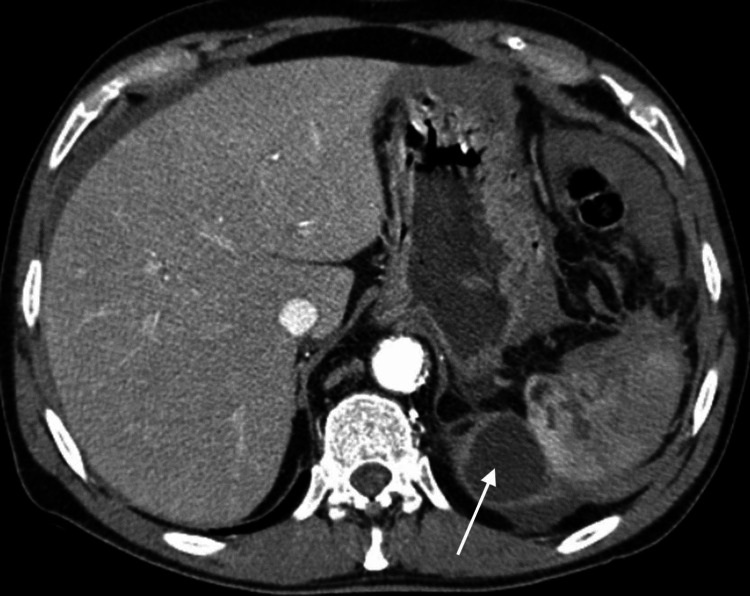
CT scan demonstrating an abdominal pancreatic collection at the anatomic site of the removed spleen (white arrow)
